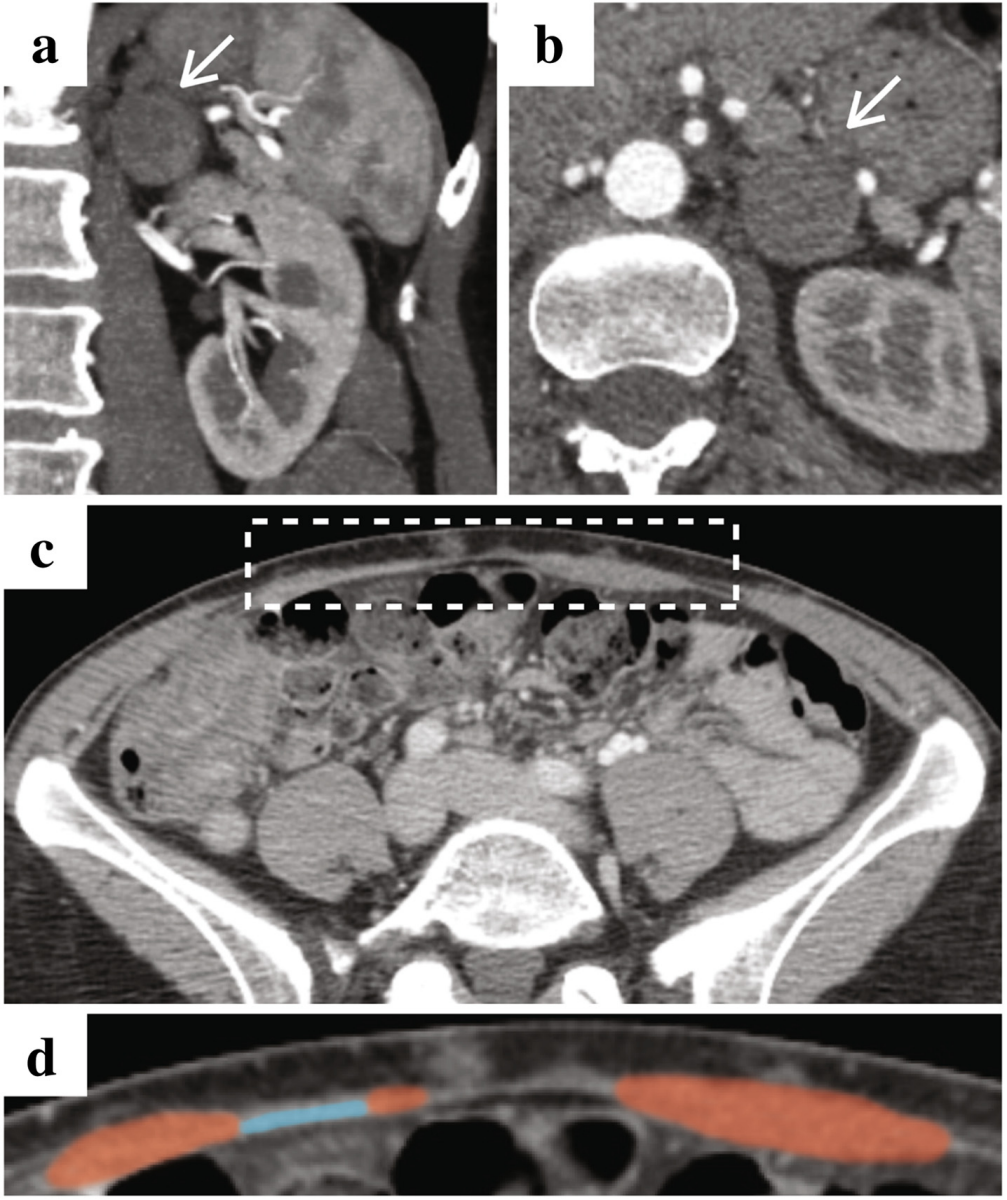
Computed Tomographic Imaging Prior to Laparoscopy Following Breast Surgery. a Coronal and b axial images demonstration left-sided pheochromocytoma. c Low-magnification axial image of the abdomen (*white box* highlights anterior abdominal wall). d High-magnification axial image of the anterior abdominal wall (*red* and *blue* indicate rectus abdominis muscle and mesh, respectively)
